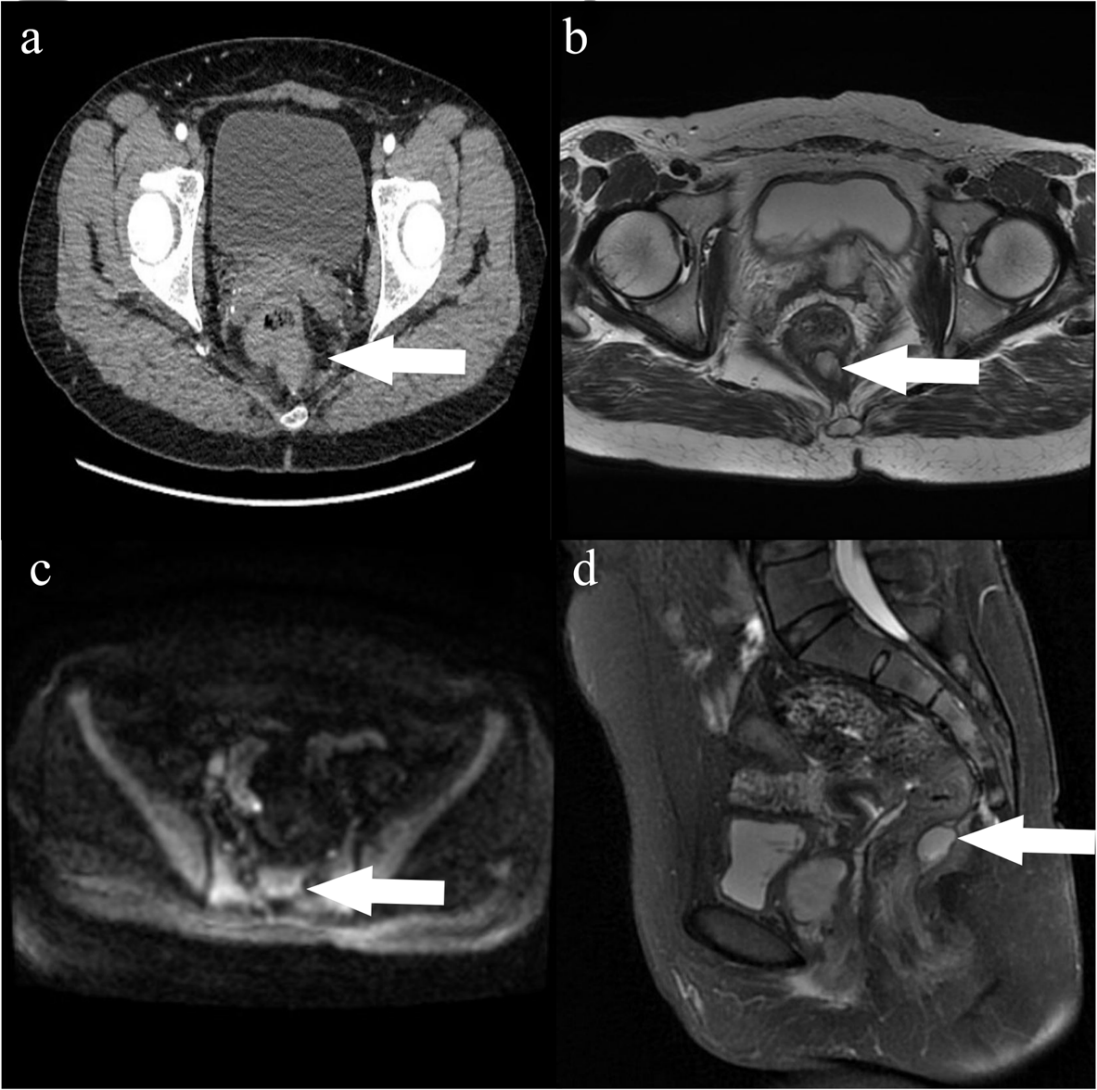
The imaging of sacrococcygeal teratoma. a and b: CT and MRI imaging demonstrated that the lesion (white arrow) located behind the rectum and in front of the coccyx. c: the lesion showed high signal on DWI (white arrow); d: T2WI fat suppression phase (sagittal position) showed that the tumor was located behind the rectum (white arrow)
